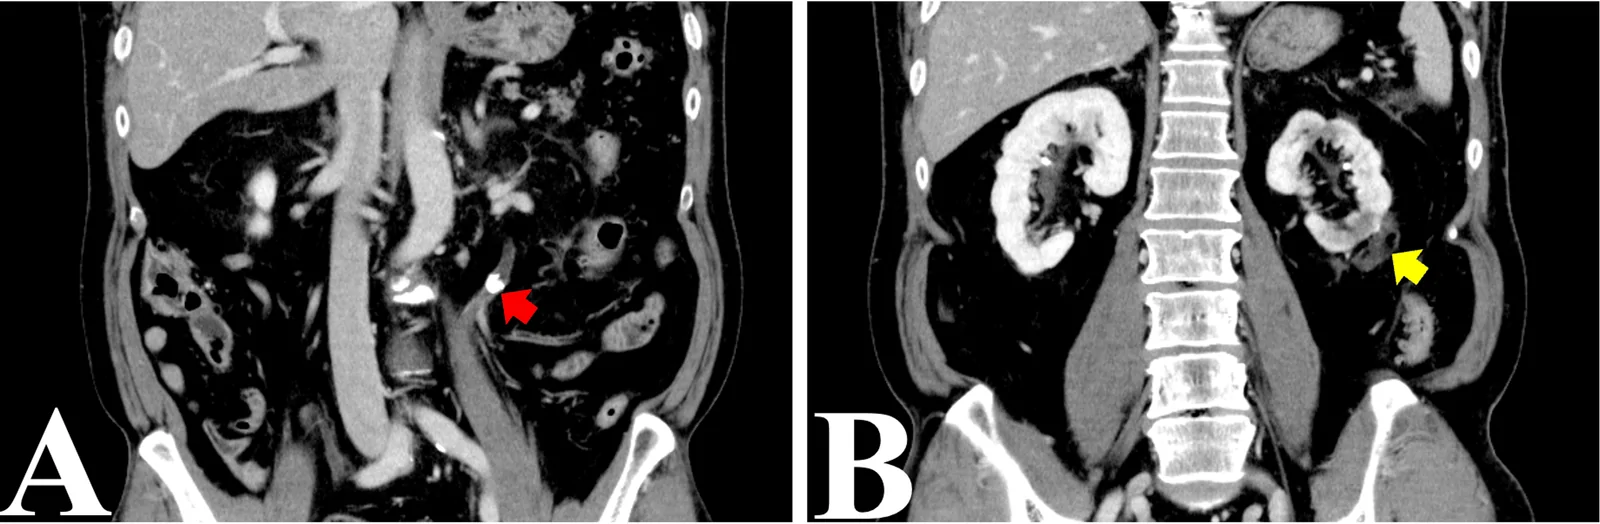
Postoperative computed tomography findings. (A) Computed tomography performed three months after robot-assisted partial nephrectomy demonstrated migration of the 9-mm calculus to the proximal ureter (red arrow). (B) Follow-up computed tomography showed no evidence of local recurrence, hemorrhage, urinary leakage, or hydronephrosis (yellow arrow).
